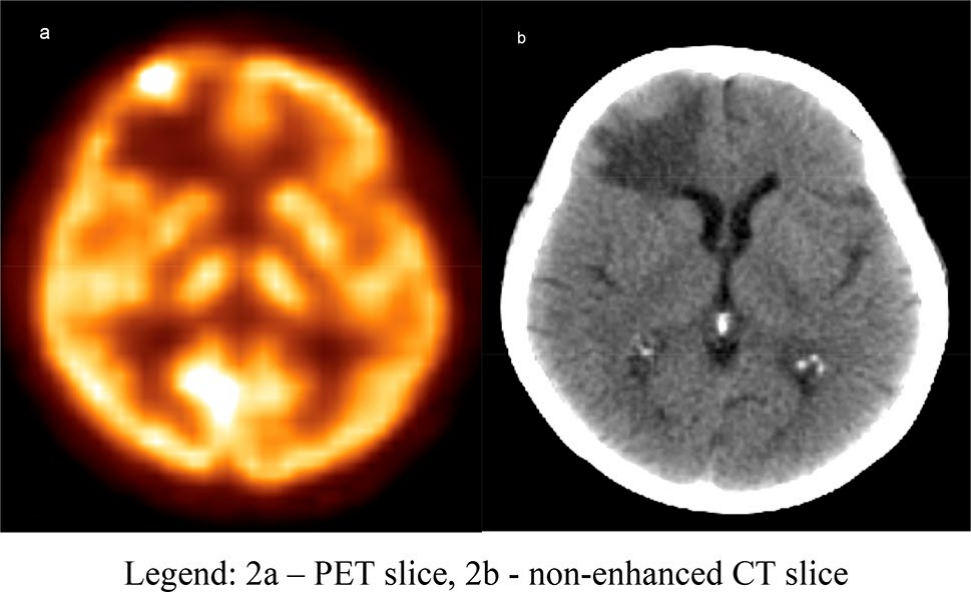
46 y.o. patient with ovarian cancer scanned with the [18F]FDG PET/CT before surgery. Abnormal finding within the right frontal lobe showing a lesion with increased [18F]FDG uptake surrounded by brain tissue oedema—ovarian cancer metastasis (*source*: original figure; software—Philips Fusion Viewer). (a) PET slice, (b) non-enhanced CT slice.
